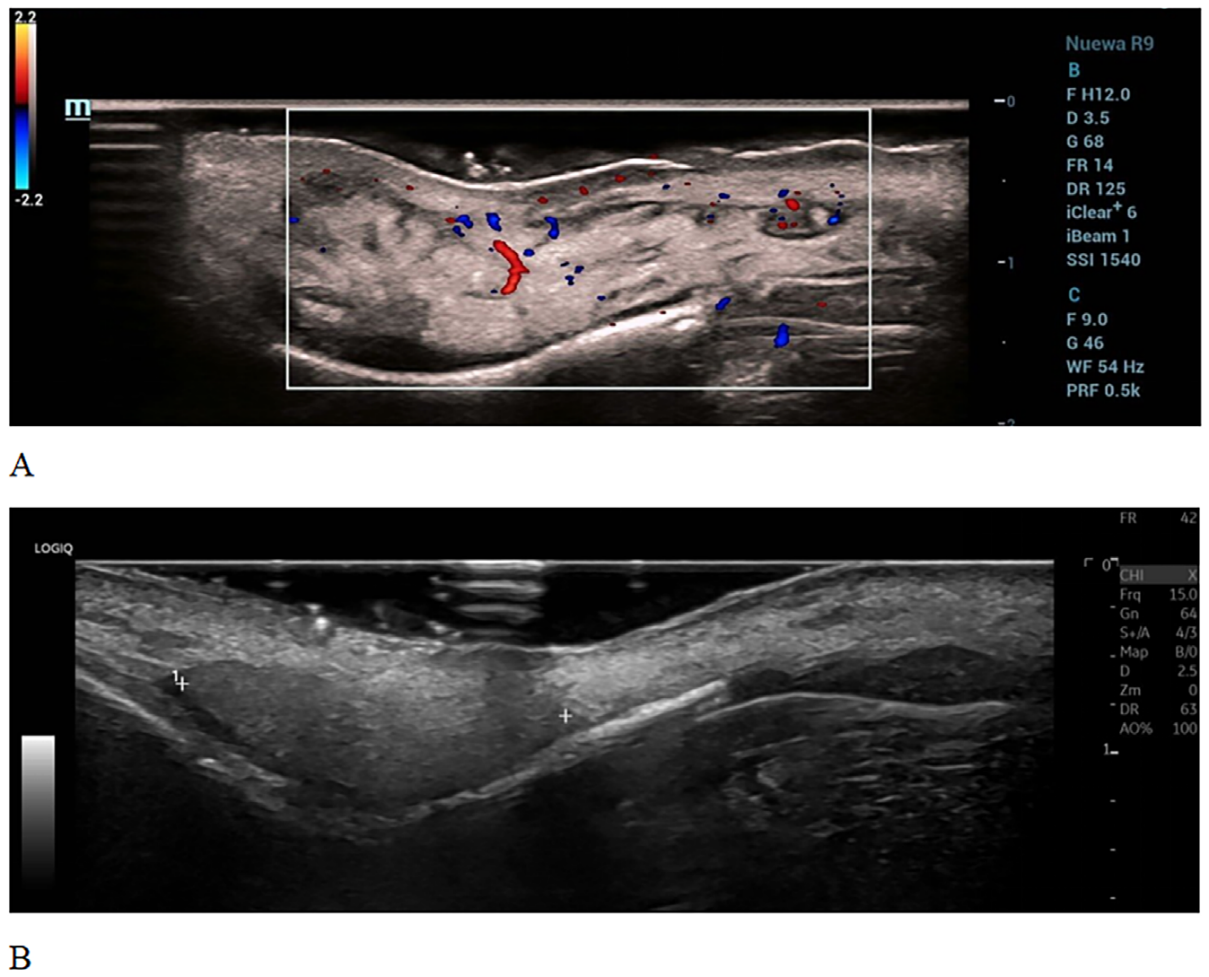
Facial color ultrasound images of Case 1. (A) Before treatment, an area of abnormal echogenicity (approximately 4.6 × 1.7 × 0.7 cm in size) is observed in the deep subcutaneous fat layer from the nasal root to the nasal dorsum. It is closely attached to the bone, with uneven internal echogenicity. A band‐like low echogenicity is visible, with point‐like blood flow seen inside and a slightly abundant blood flow visualized around it. (B) After 14 days of treatment, an area of high echogenicity (approximately 2.0 × 1.2 × 0.6 cm in size) is observed in the deep subcutaneous fat layer from the nasal root to the lower part of the nasal dorsum. It is closely attached to the bone, with moderately uniform internal echogenicity. The boundary with the surrounding tissue is partially unclear, and the band‐like boundary is weakened. It is located approximately 0.10 cm beneath the surface, mainly on the upper part of the hump. No obvious blood flow is visible inside, but a small amount of blood flow is visible around it. The abnormal area and blood flow are reduced as compared to those observed during the pretreatment examination.
